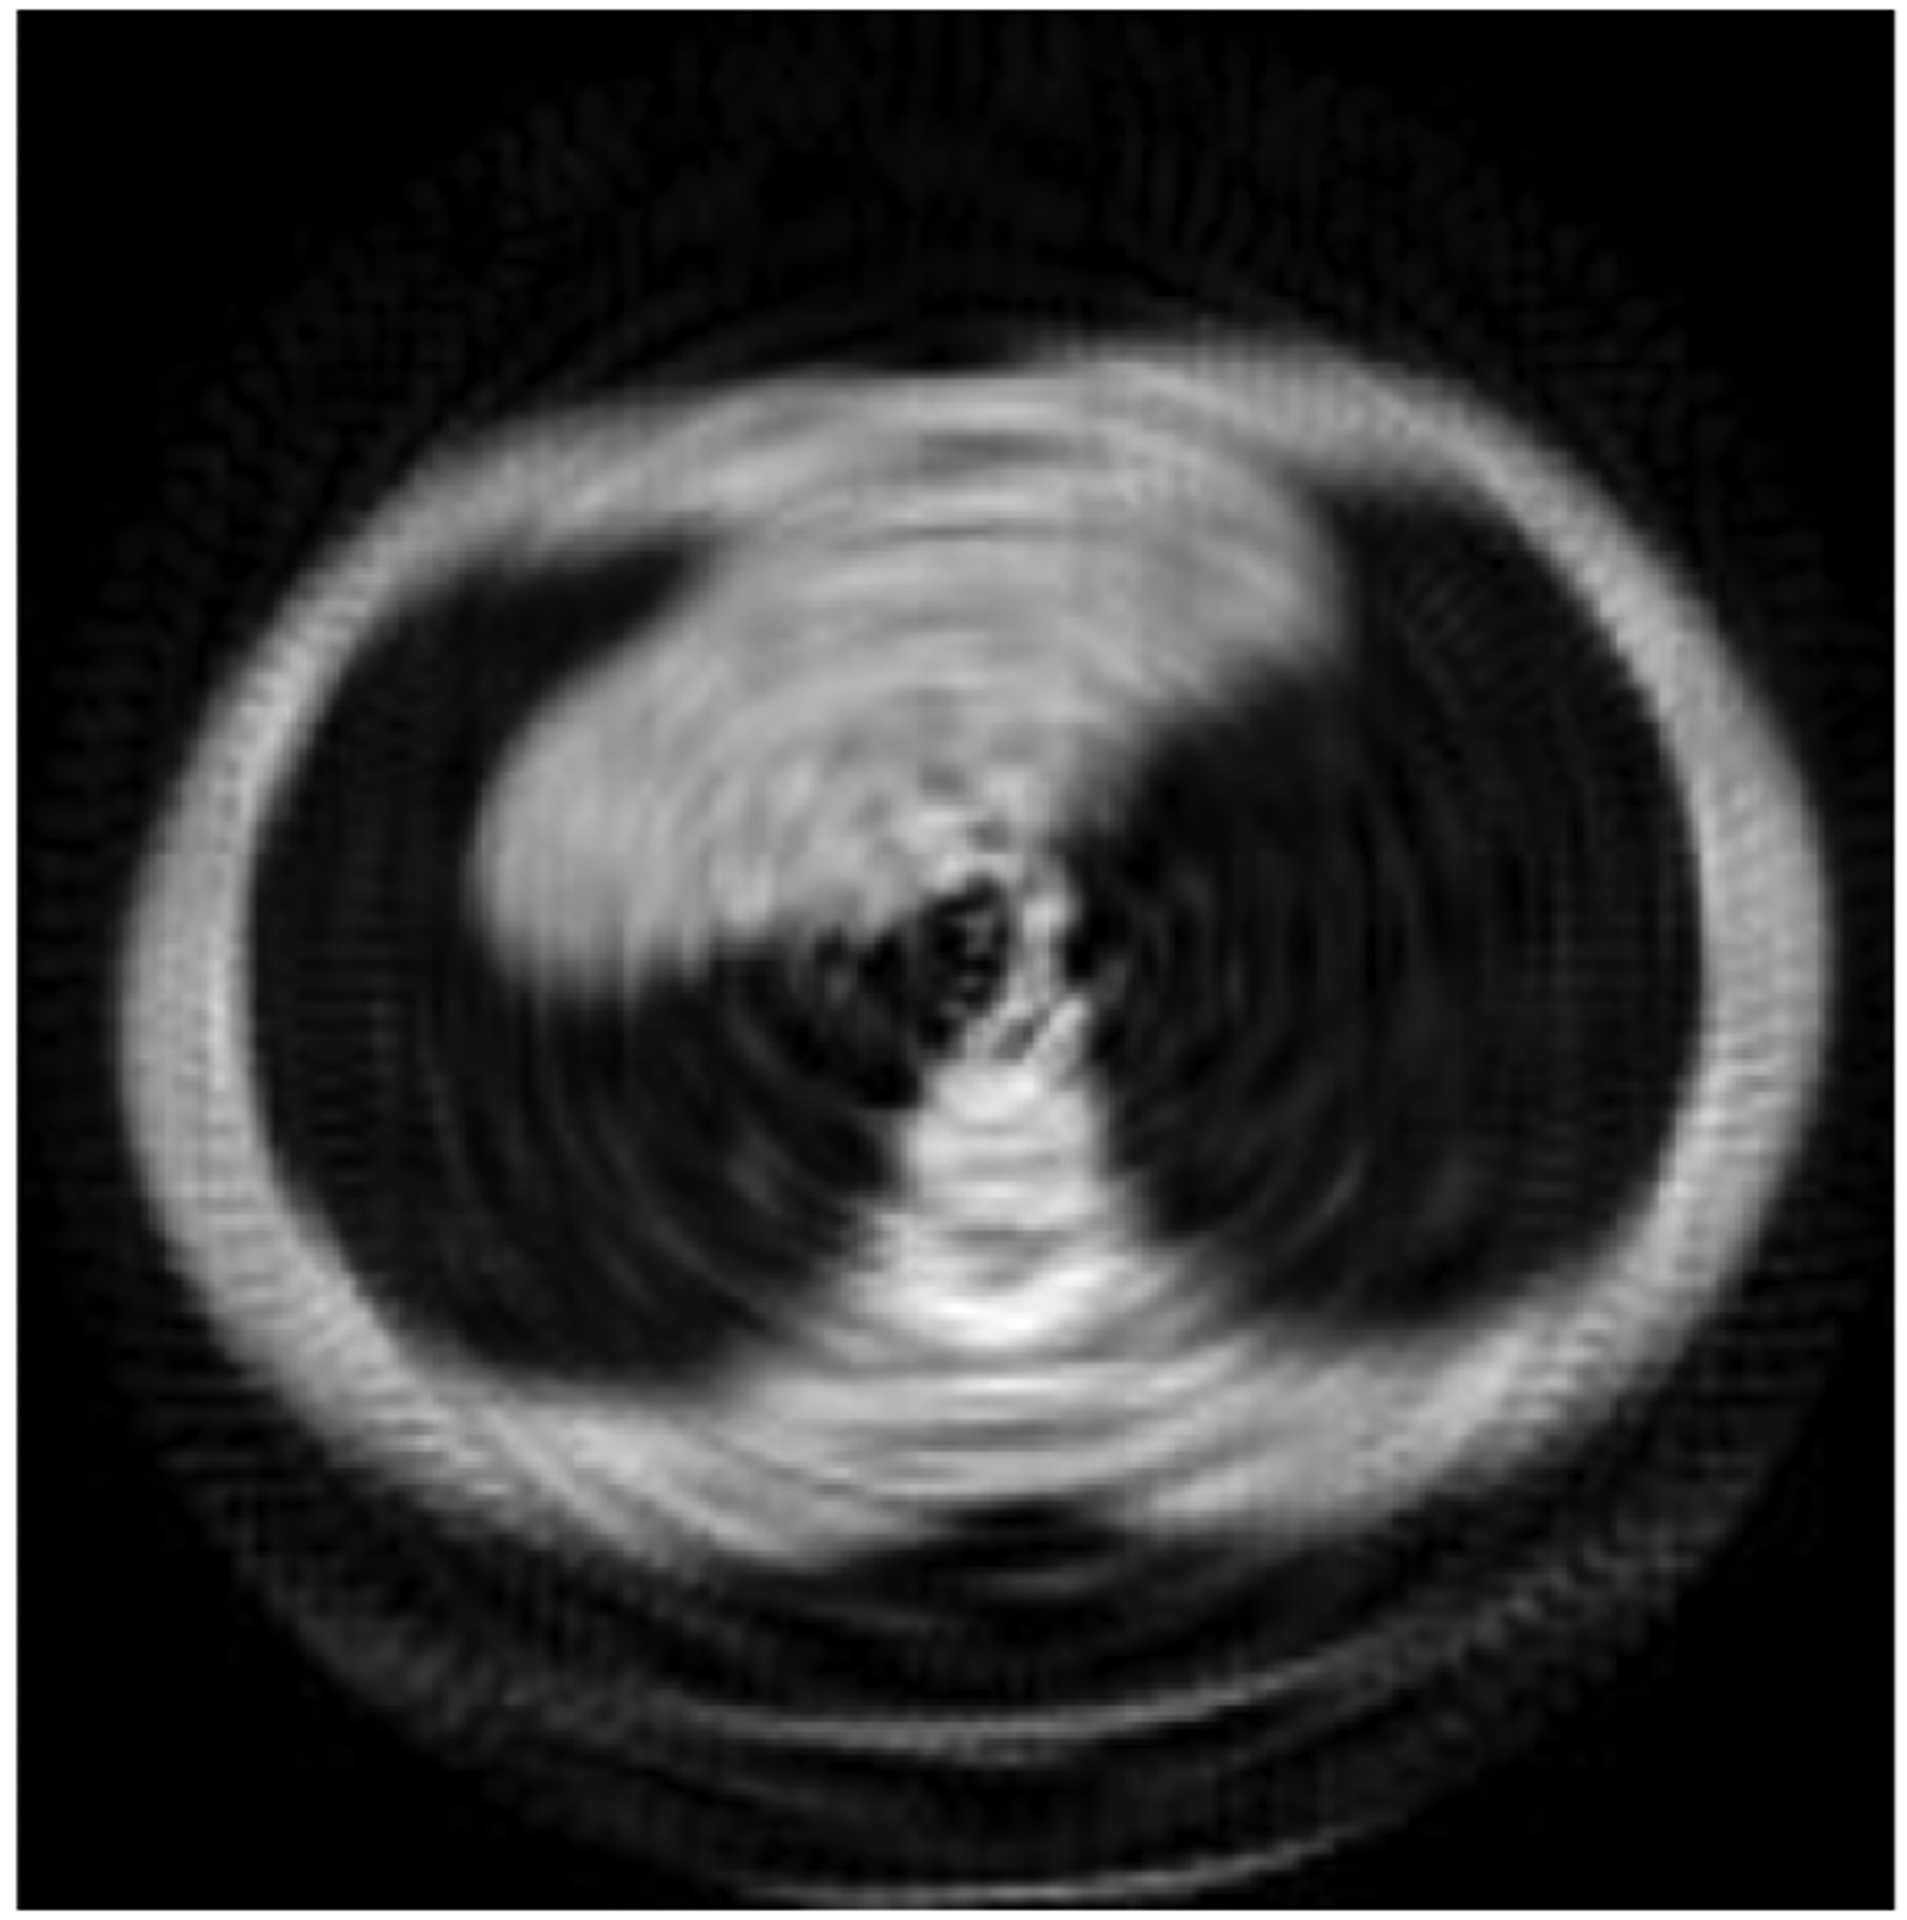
Reconstruction from linear-interpolated sonogram.
